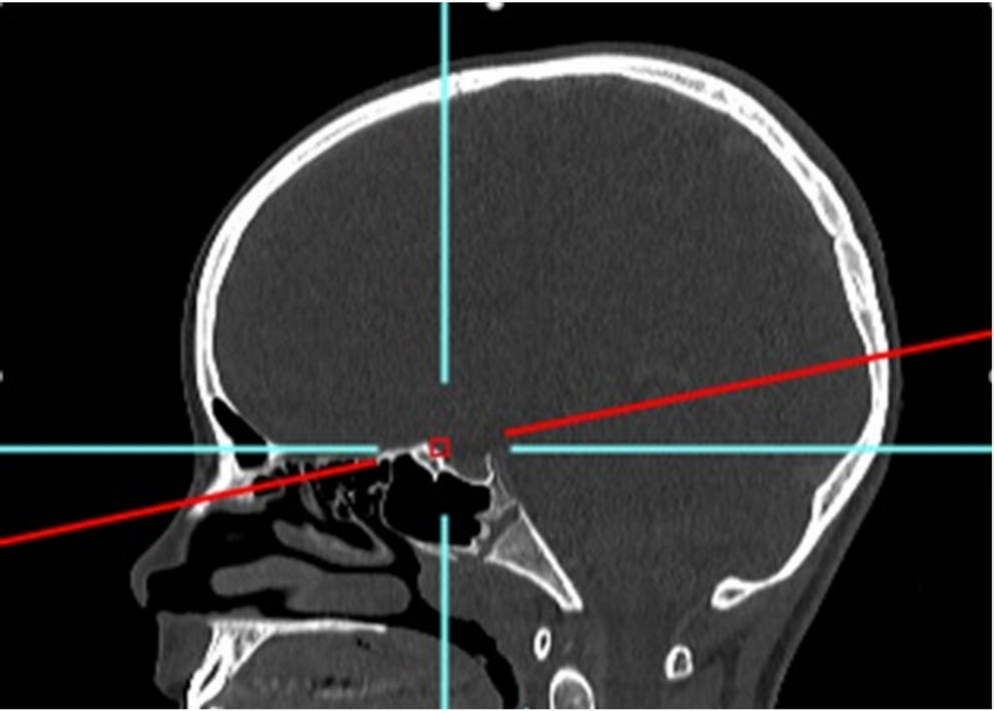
Craniofacial CT-scan, sagittal view. The red line represents the level of neuro-ocular plane as described by Cabanis et al. [25, 26]
